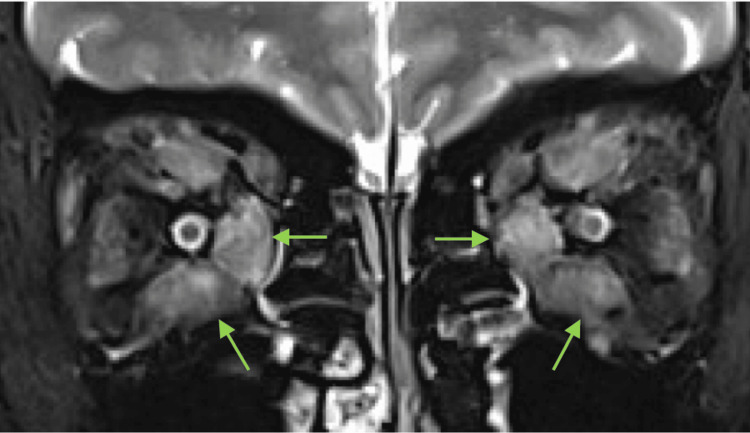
MRI findings of Graves’ orbitopathy with dysthyroid optic neuropathy (T2-weighted and fat-suppressed images using short-tau inversion recovery sequences). Arrows indicate bilateral muscle enlargement, with high signal intensity and more prominent inferior and medial rectus muscles.
